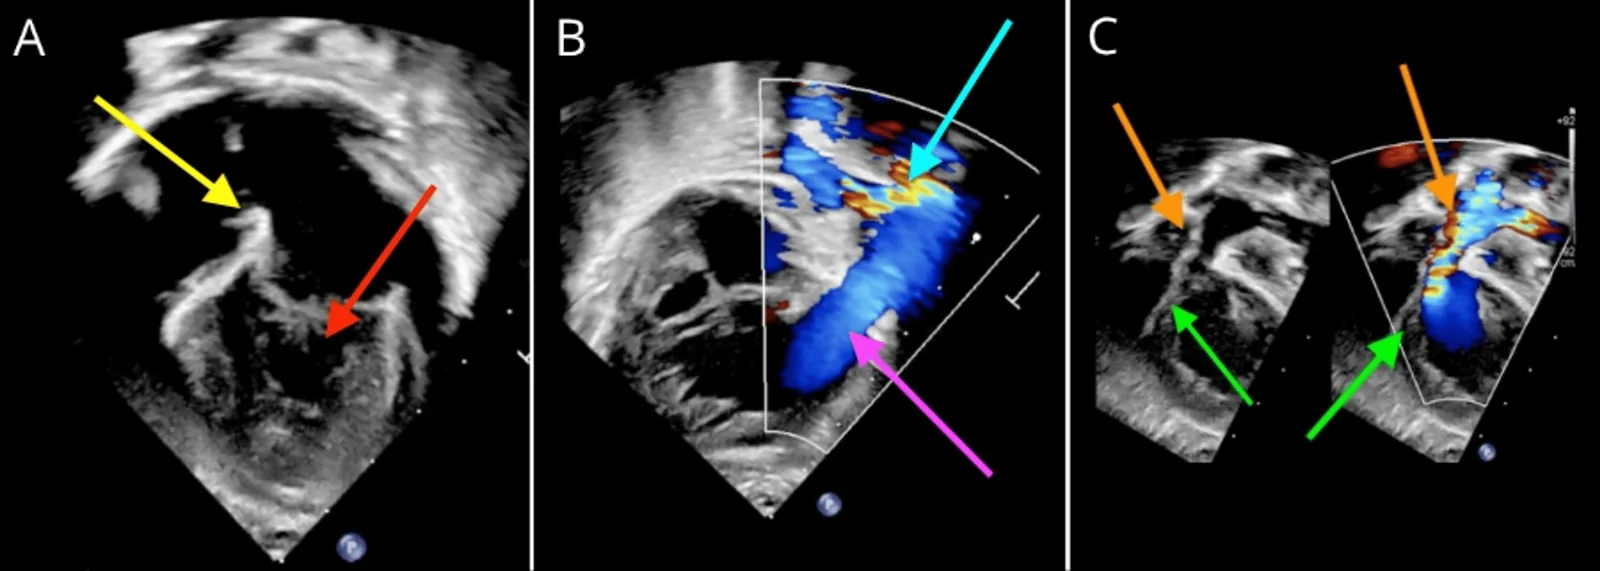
Transthoracic echocardiogram demonstrating tricuspid atresia and single‑ventricle physiology. A) Apical four-chamber view showing an atretic tricuspid valve (yellow arrow) and hypoplastic right ventricle (red arrow). B) Color Doppler imaging demonstrating flow across a large ventricular septal defect (blue arrow) from the dominant left ventricle to the diminutive right ventricular outflow (pink arrow). Arrows indicate the turbulent jet across the septal defect. C) Additional apical view emphasizing single-ventricle morphology (orange arrows) and rudimentary right ventricular component (green arrows).
